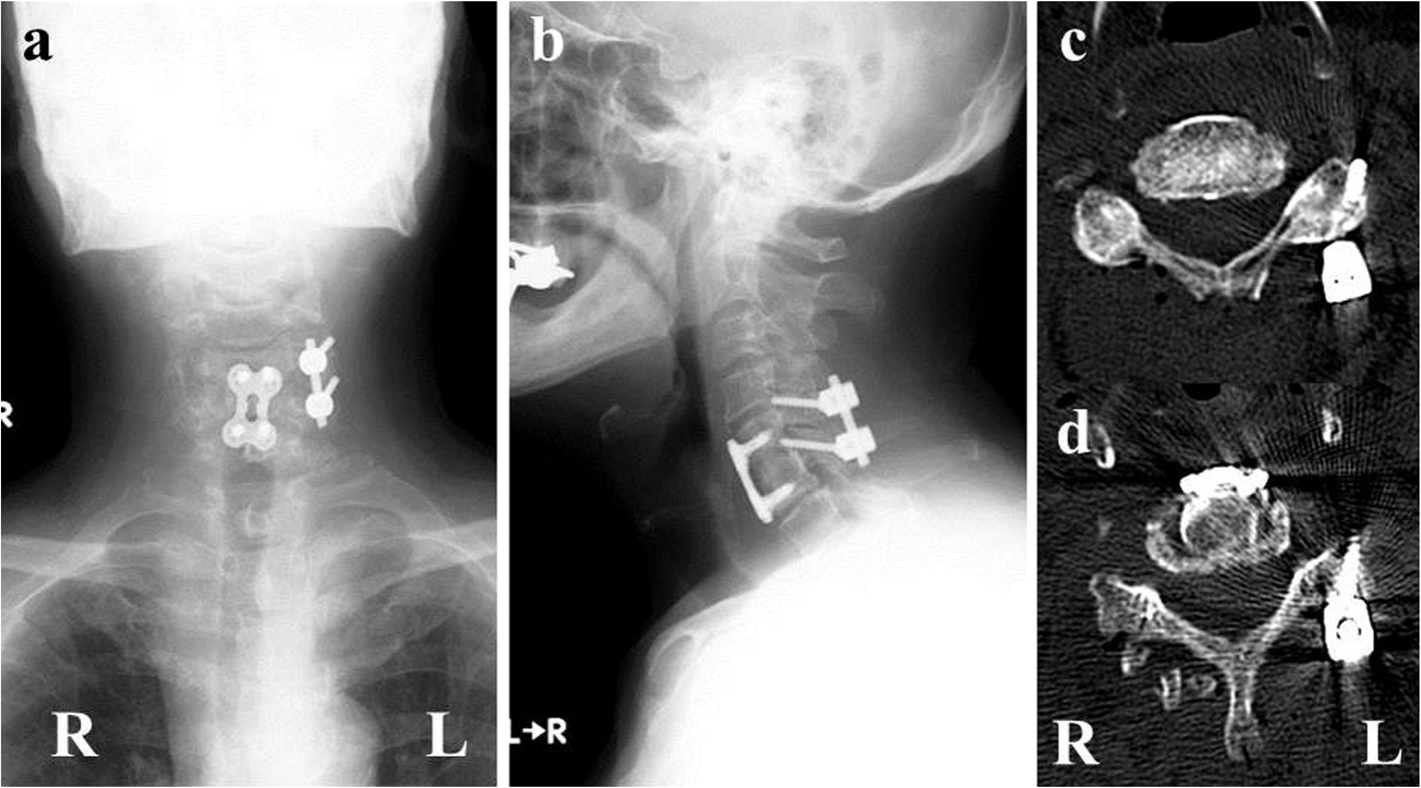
Plain X-ray imaging and computed tomography scans obtained six months after surgery. We performed surgery to remove a part of both sides of the facet joints at the C5 to C6 joint, and posterior fusion with lateral mass screws on the left side. After posterior fusion, anterior fusion using a plate and screws was performed. (a) X-ray anterior-posterior view; (b) X-ray lateral view; (c) computed tomography scan of C5; (d) computed tomography scan of C6. R: right side. L: left side.
